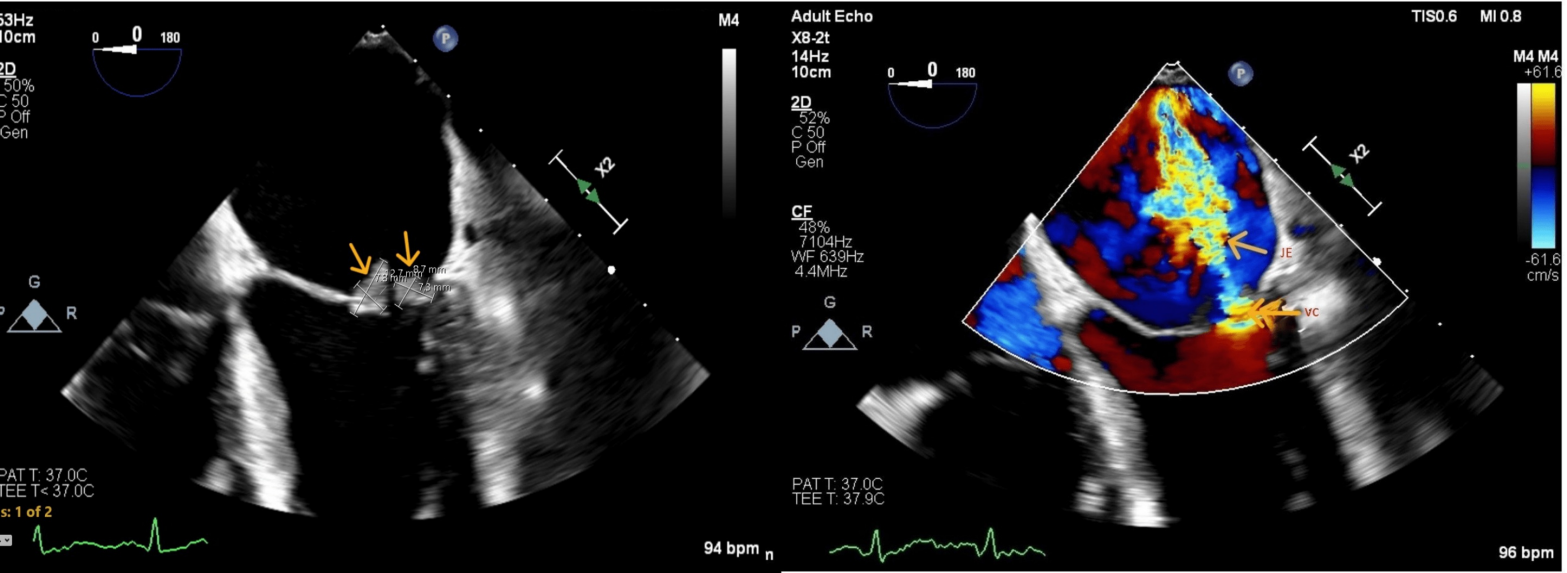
Transesophageal echocardiogram (mitral valve view) Arrows indicate mitral valve leaflet vegetations (left panel). Additional arrows highlight vena contracta (VC) and jet expansion (JE) on color flow Doppler, demonstrating mitral regurgitation (right panel).
